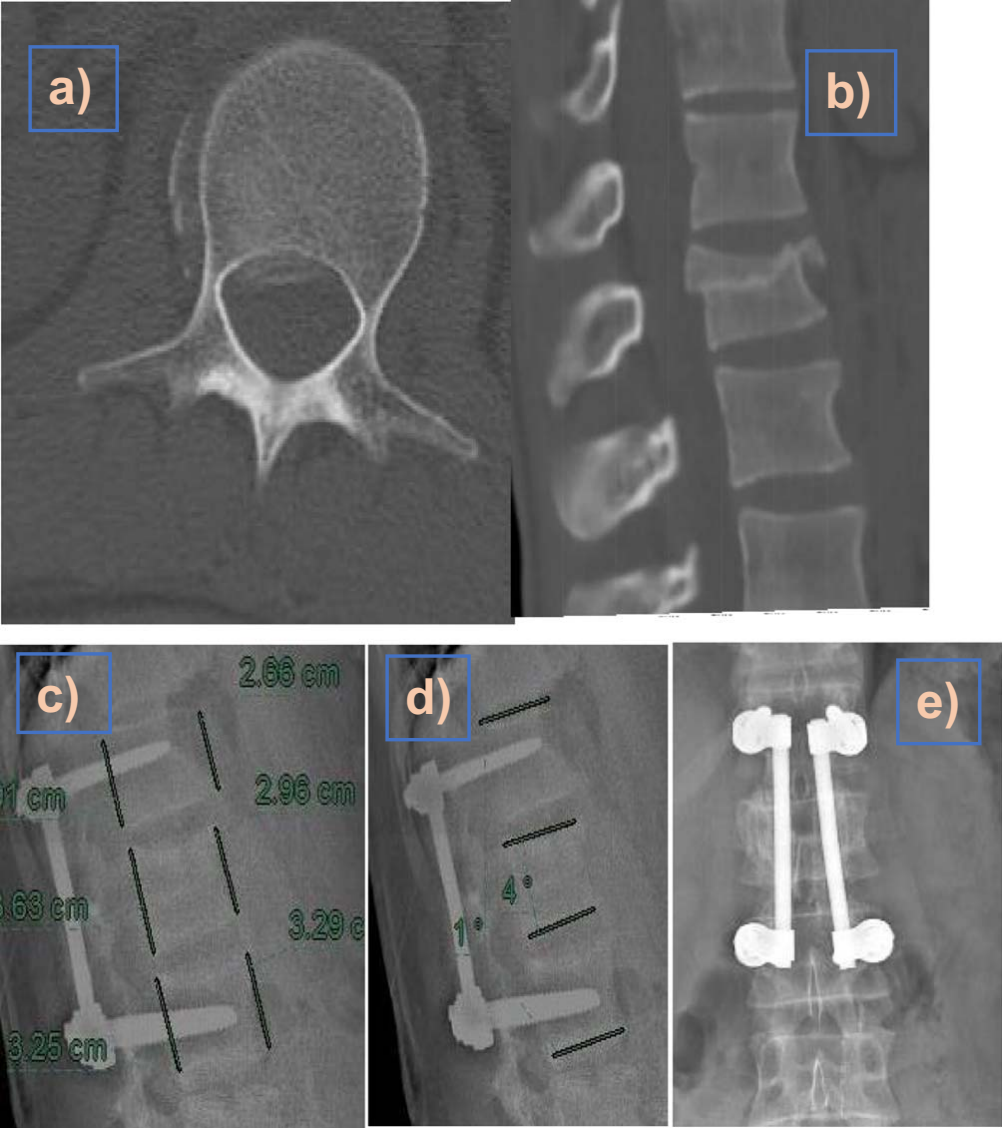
36-year-old male patient after RTA with a burst fracture of L1 (AO type A3) and associated bilateral calcaneal fracture with Frankle grade E. Patient was operated on the fifth day after trauma using USS. (a, b) Axial and sagittal CT scan delineating the burst fracture at L1; (c–e) lateral and AP X-rays after one-year follow-up showing good preservation of the vertebral height and sagittal angular alignment.
